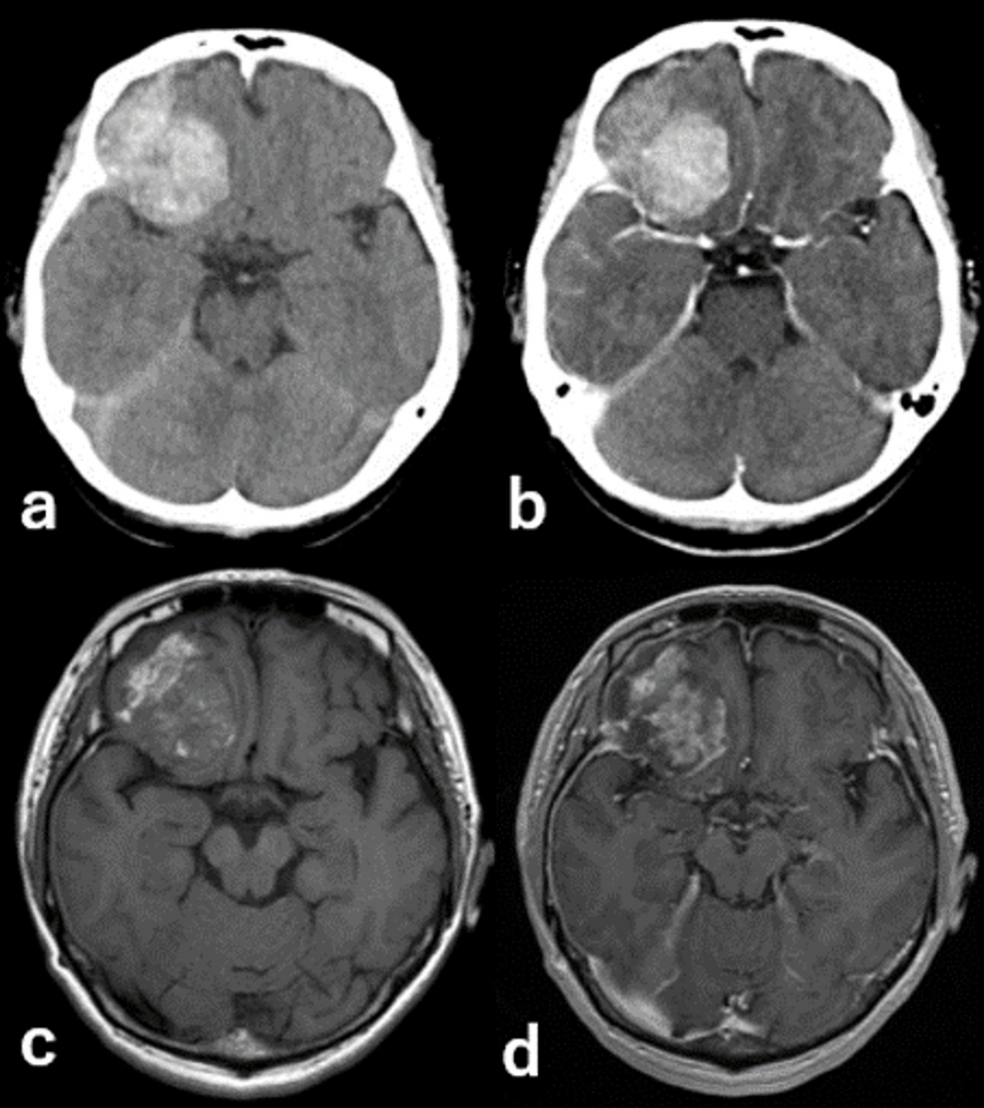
Imaging findings - (a) plane CT (b) enhanced CT (c) T1 (d) enhanced MRI CT: computed tomography; MRI: magnetic resonance imaging
